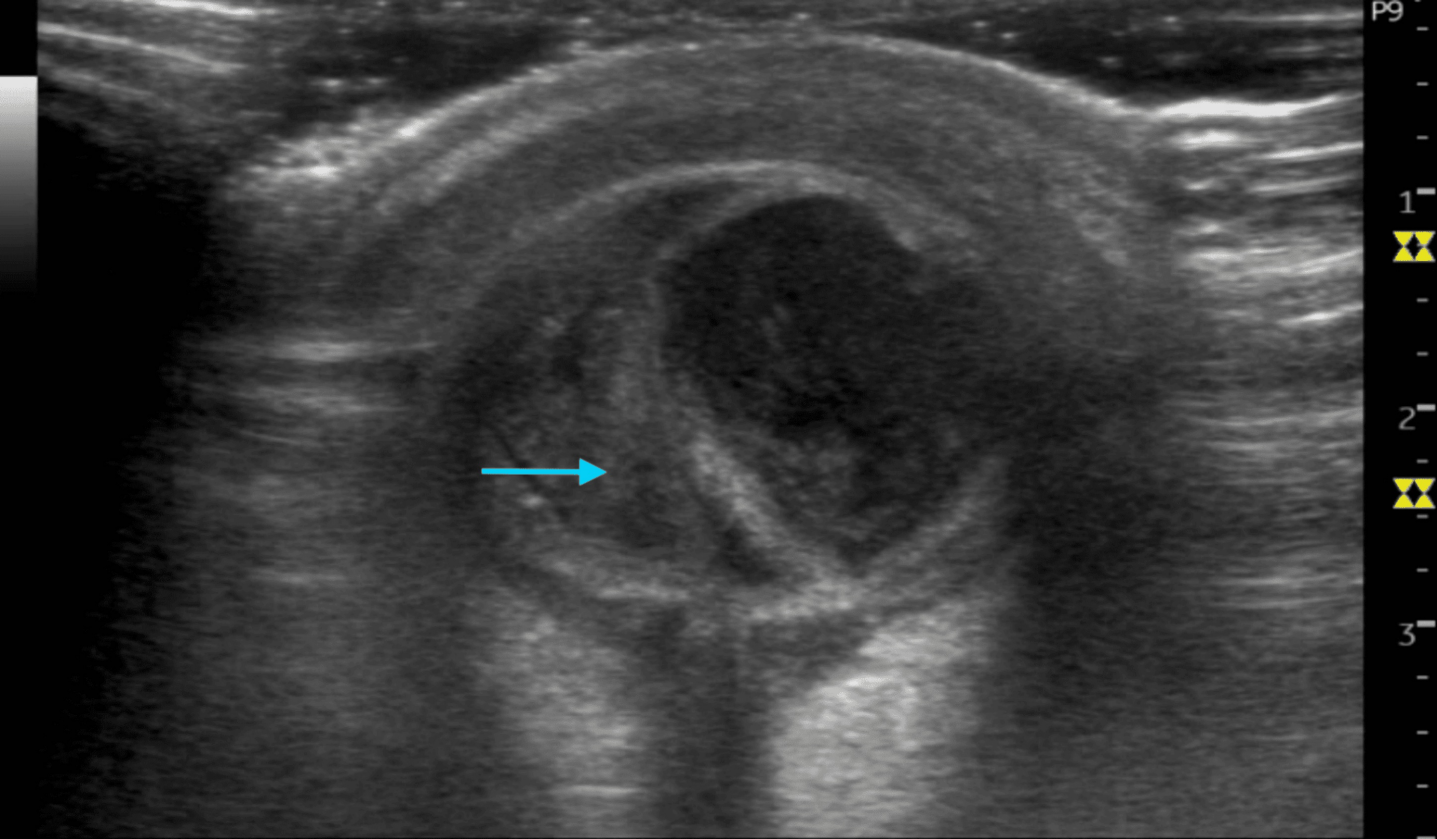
Intraocular debris seen on ocular POCUS of the left eye as depicted by an arrow. POCUS: point-of-care ultrasound
